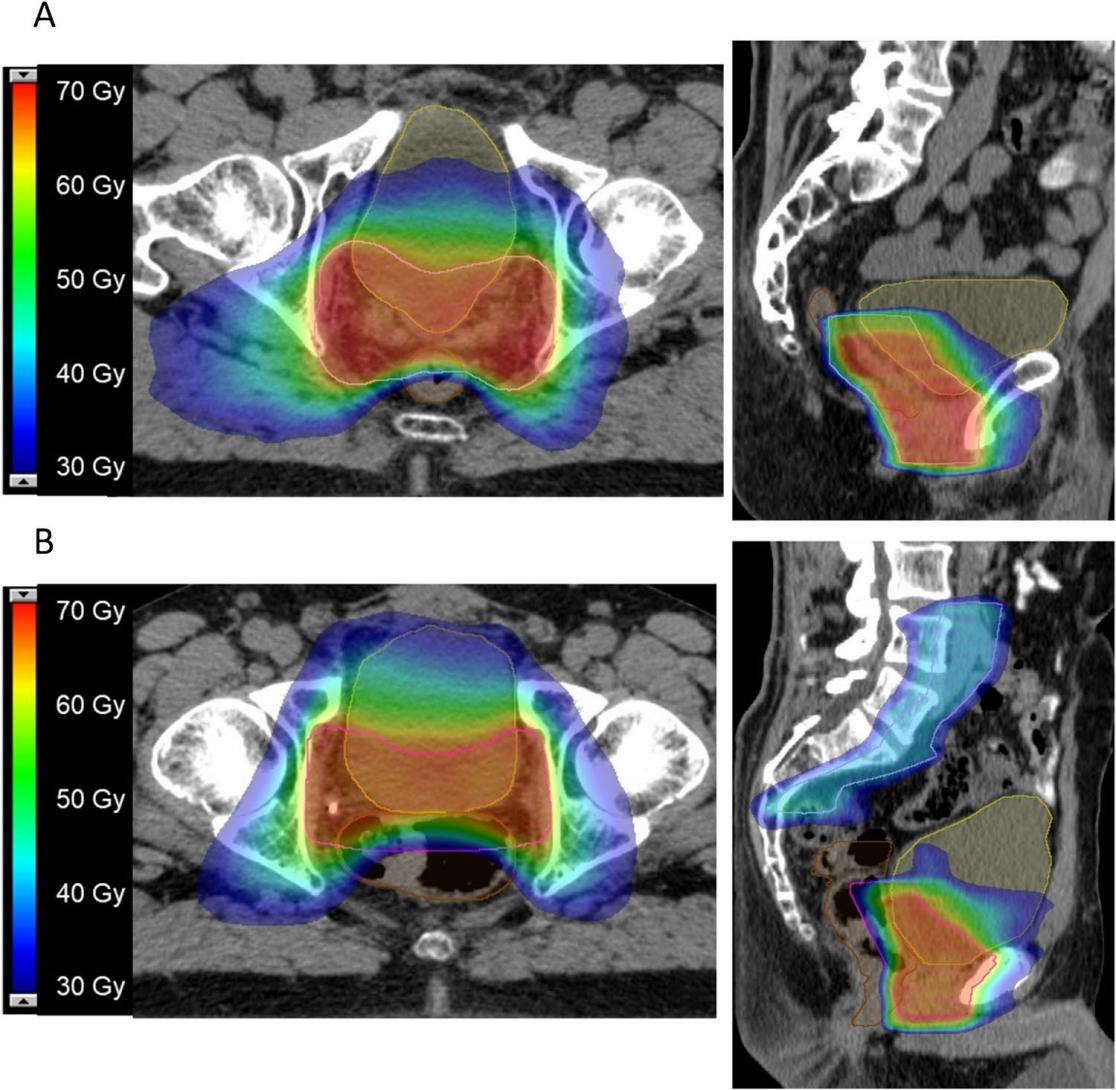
Case reports. This figure shows the treatment plans of two exemplary patients on axial and sagittal planning CT slides. The planning target volume is shown in pink, the rectum in brown, and the bladder in yellow. Radiotherapy isodoses are shown in colorwash according to the legend. Patient A: the patient presented with the following clinical characteristics after definitive prostatecomy: pT2b pN0 (0/26), L0 V0 Pn1 R1, ISUP 3, iPSA 8.5 ng/ml. Before sRT, mpMRI and PSMA-PET did not show any suspicious lesions within or outside the prostate bed. PSA prior to sRT was 0.18 ng/ml. sRT was delivedered to the prostate bed in 1.8 Gy per fraction up to 66.6 Gy. At 44 months of follow-up, no recurrent disease could be observed. Patient B: the patient presented with the following clinical characteristics after definitive prostatectomy: pT3b pN1 (3/25), L1 V0 Pn1 R1, ISUP 3, IPSA 13.8 ng/ml. Before sRT, mpMRI and PSMA-PET did not show any suscipious lesions within or outside the prostate bed. sRT was deleivered to the prostate bed in 1.8 Gy per fraction up to 66.6 Gy. Elective pelvis were treated with 1.8 Gy per fraction up to 45 Gy. Regions with positive lymph nodes were escalated with boost of 1.8 Gy per fraction up to 54 Gy. Androgen deprivation therapy was given for 6 months. After 31 months, the patient experienced a biochemical recurrence. PSMA-PET/CT imaging revealed a bone metastasis in the breast vertebrae 7
